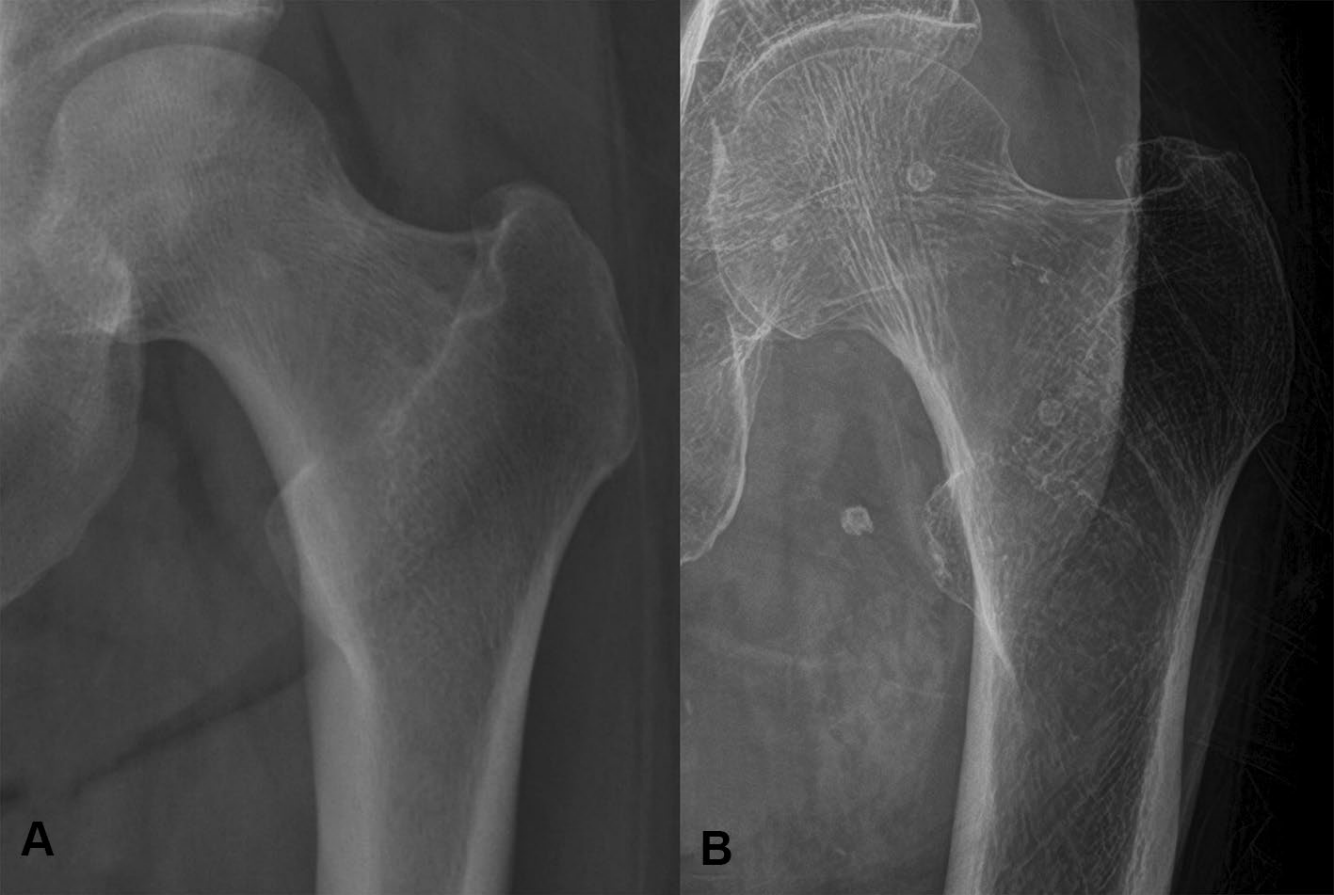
The comparison between normal hip radiographs and osteoporosis. Normal hip radiograph of a 57-year woman, with confirmed T-score of − 0.7, is shown in (A), and the hip radiograph of 84-year woman who was diagnosed with osteoporosis with T-score of − 4.6 is shown in (B).
